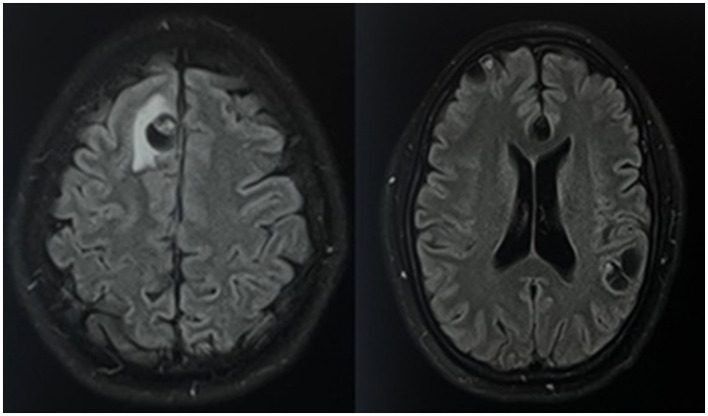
Non-contrast brain MRI – sagittal T1-weighted sequence.
